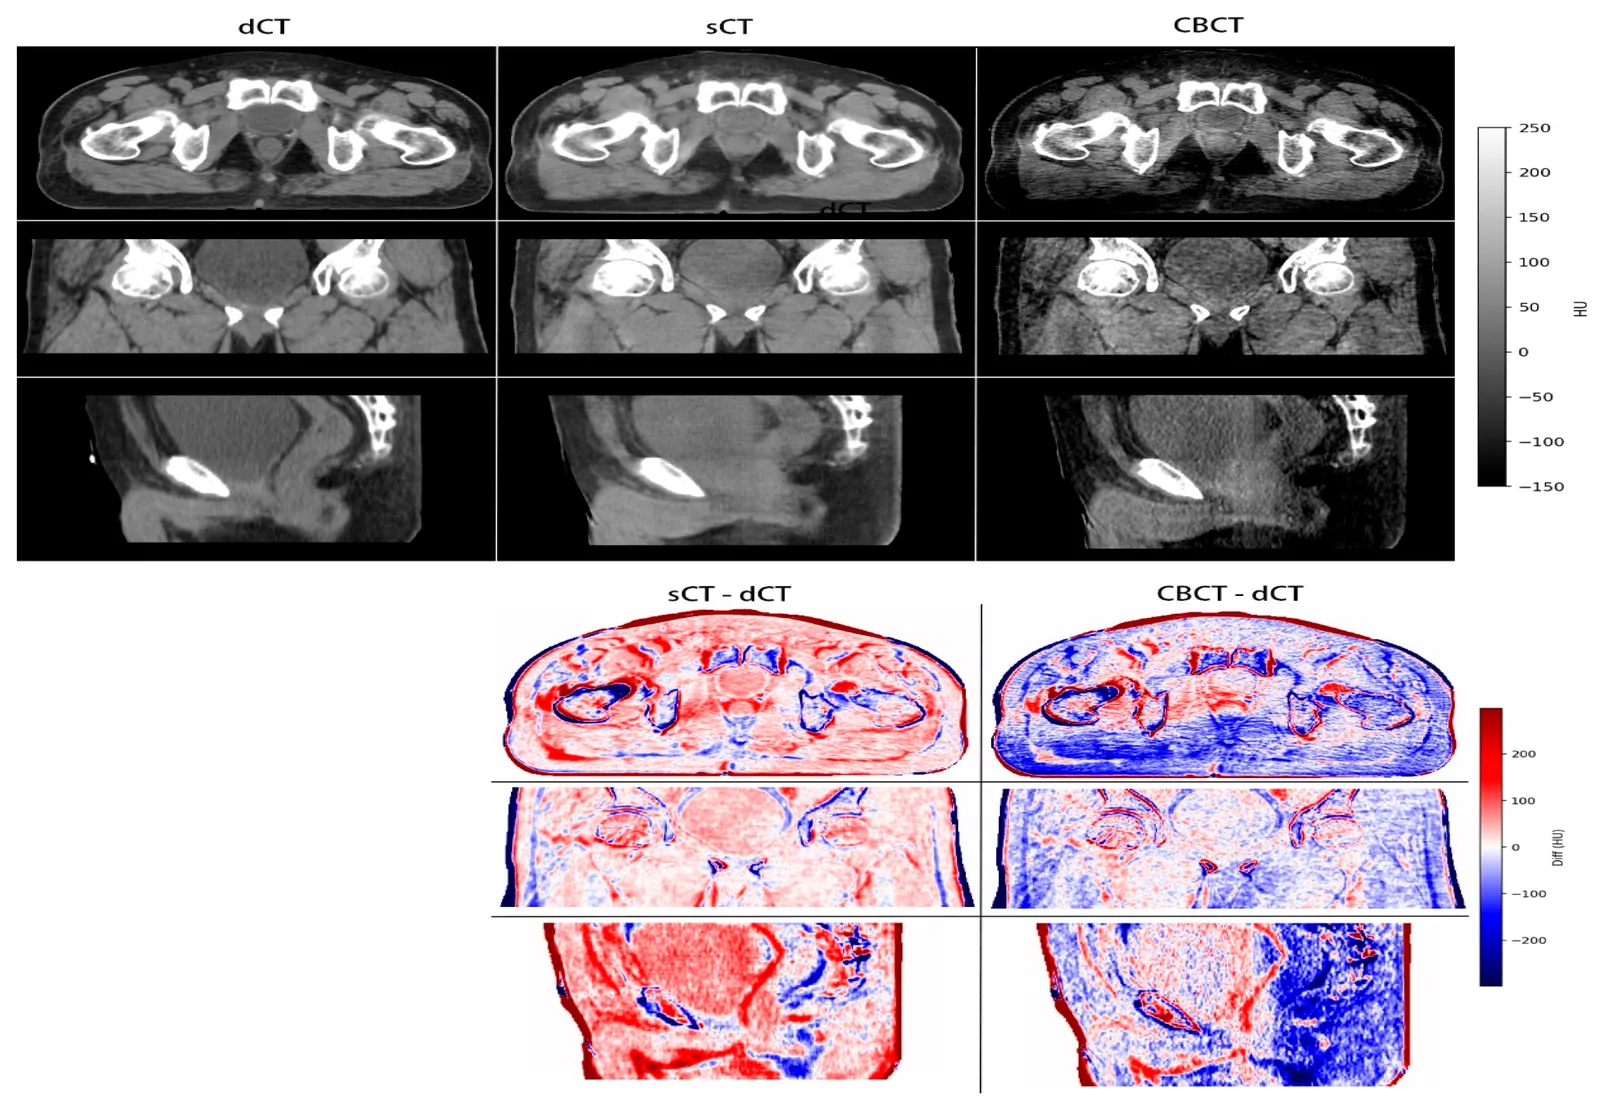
CBCT, sCT, and deformed CT images and HU difference in a prostate cancer patient. Compared with CBCT, the sCT images show markedly improved quality, with reduced noise and artifacts, and demonstrate closer consistency with the deformed CT reference standard.
